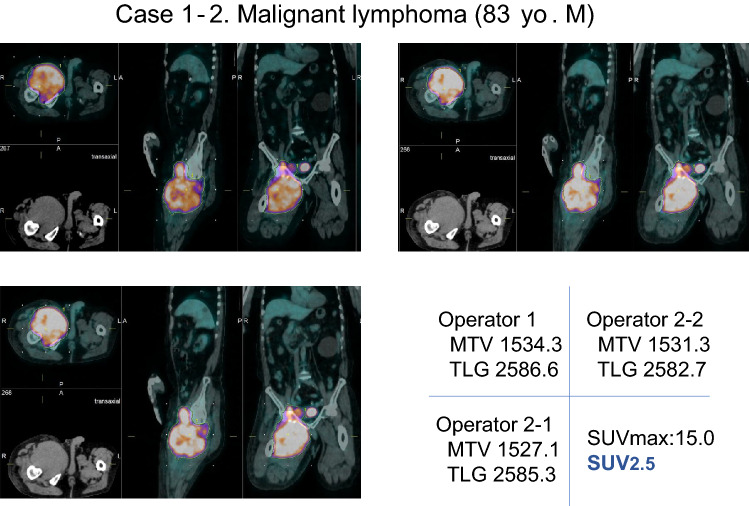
When the lower limit by the fixed value SUV2.5 was used, the variability of MTV and TLG among inter- and intra-operators was smaller than cases 30% value of SUVmax was used as lower limit
